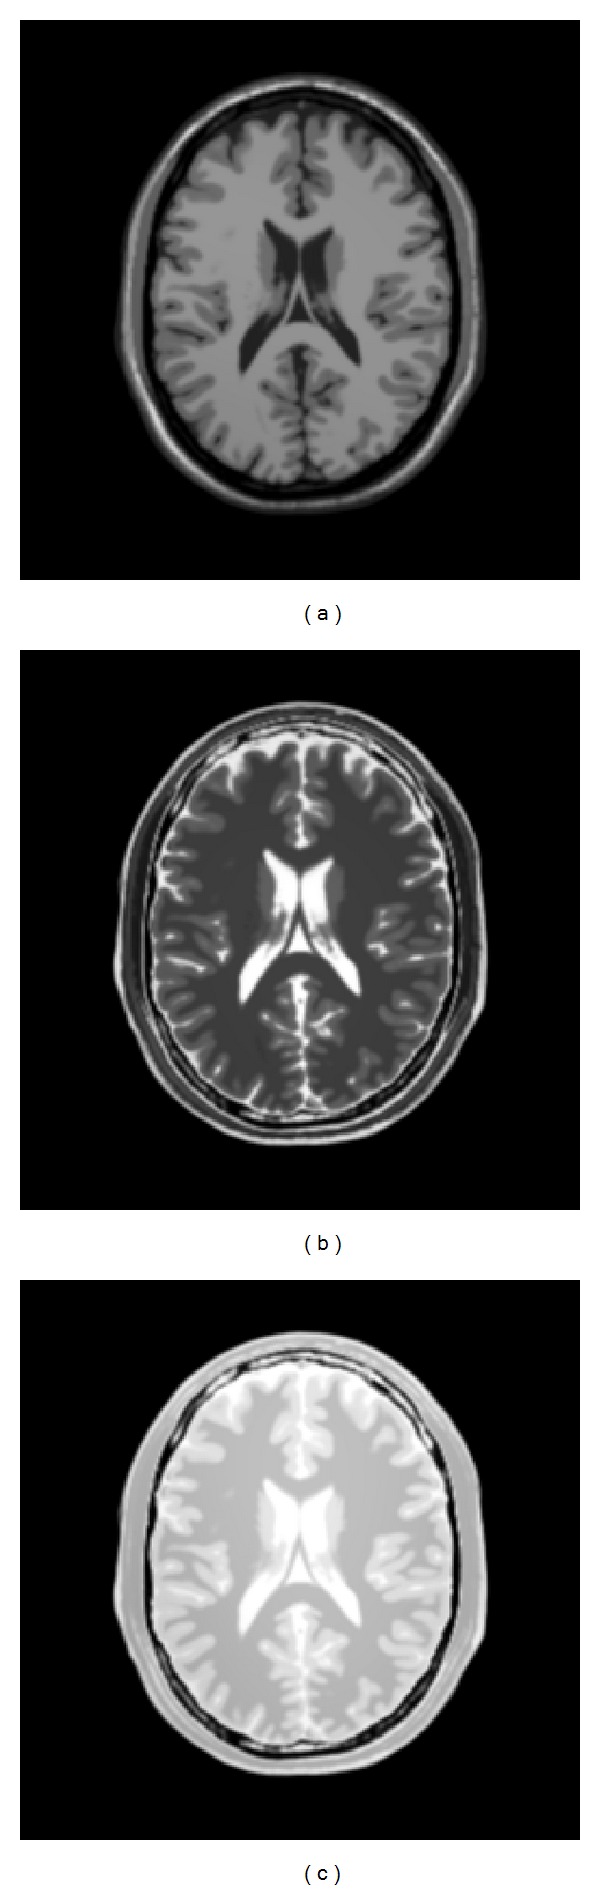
Brain MR images with three sequences volumes T1, T2, and DP [41].
